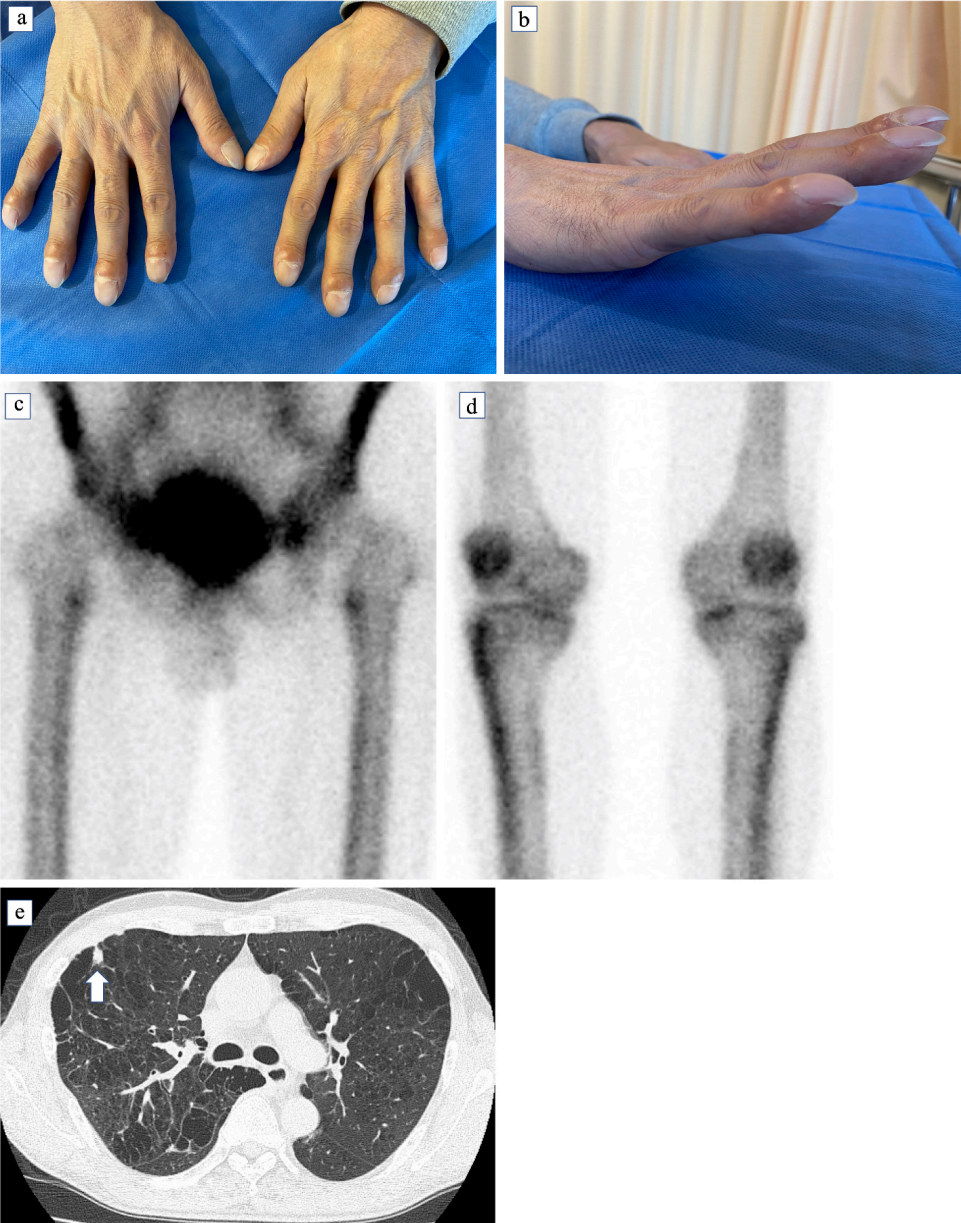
(a), (b): Clubbing of the fingers. (c), (d): Bone scintigraphy revealing areas of technetium 99 m-labeled methylene diphosphonate uptake in the femoral (c) and tibial bones (d). (e): Chest computed tomography showing a nodule in the upper lobe of the right lung.
